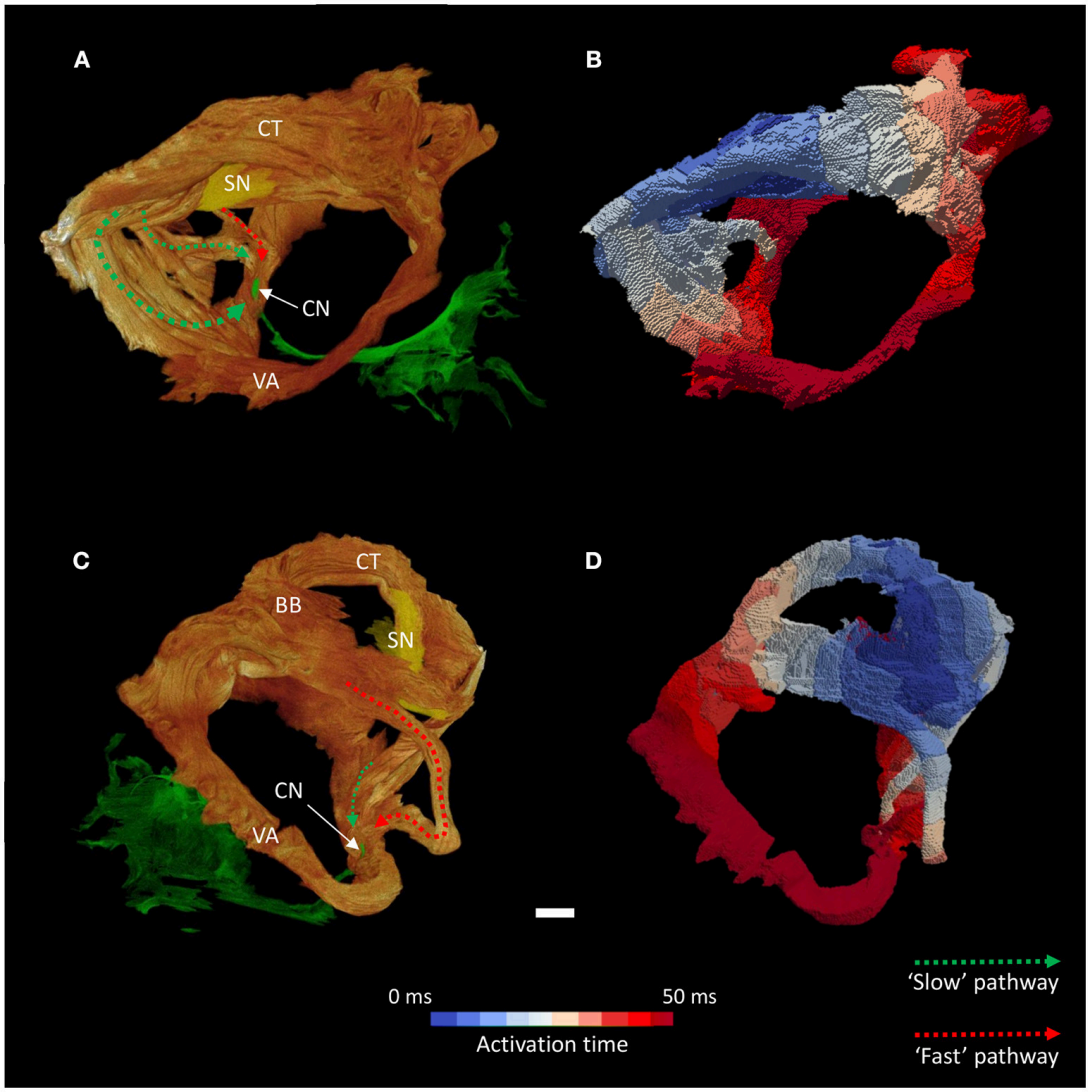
Inter-nodal conduction through the atrial muscle bundles I. Volume renderings of the atrial muscle bundles in right lateral (A) and left lateral (C) views, the normal location and direction of the “slow” pathway (green), and the septal aspect of the elongated “fast” pathway (red) are indicated by dotted arrows. (B,D) Display the corresponding electrical activation maps, showing excitation of the distal aspect of the region normally associated with the “slow” pathway precedes that of the “fast” pathway. See methods for modeling parameters. BB, Bachmann's bundle; CN, compact atrioventricular node; CT, terminal crest; SN, sinus node; VA, valve annulus. Scale bar represents 3 mm.
